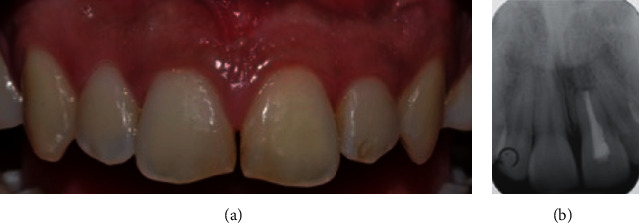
(a) Clinical and (b) radiographic view 4 years after the surgical therapy.
